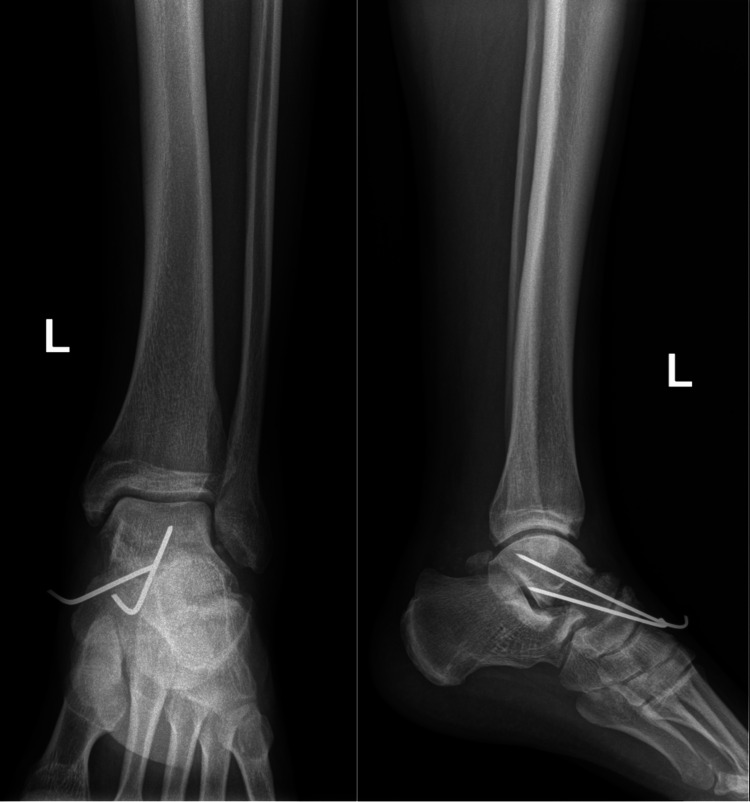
Anteroposterior (AP) and lateral view X-rays of the left ankle six weeks post-injury
